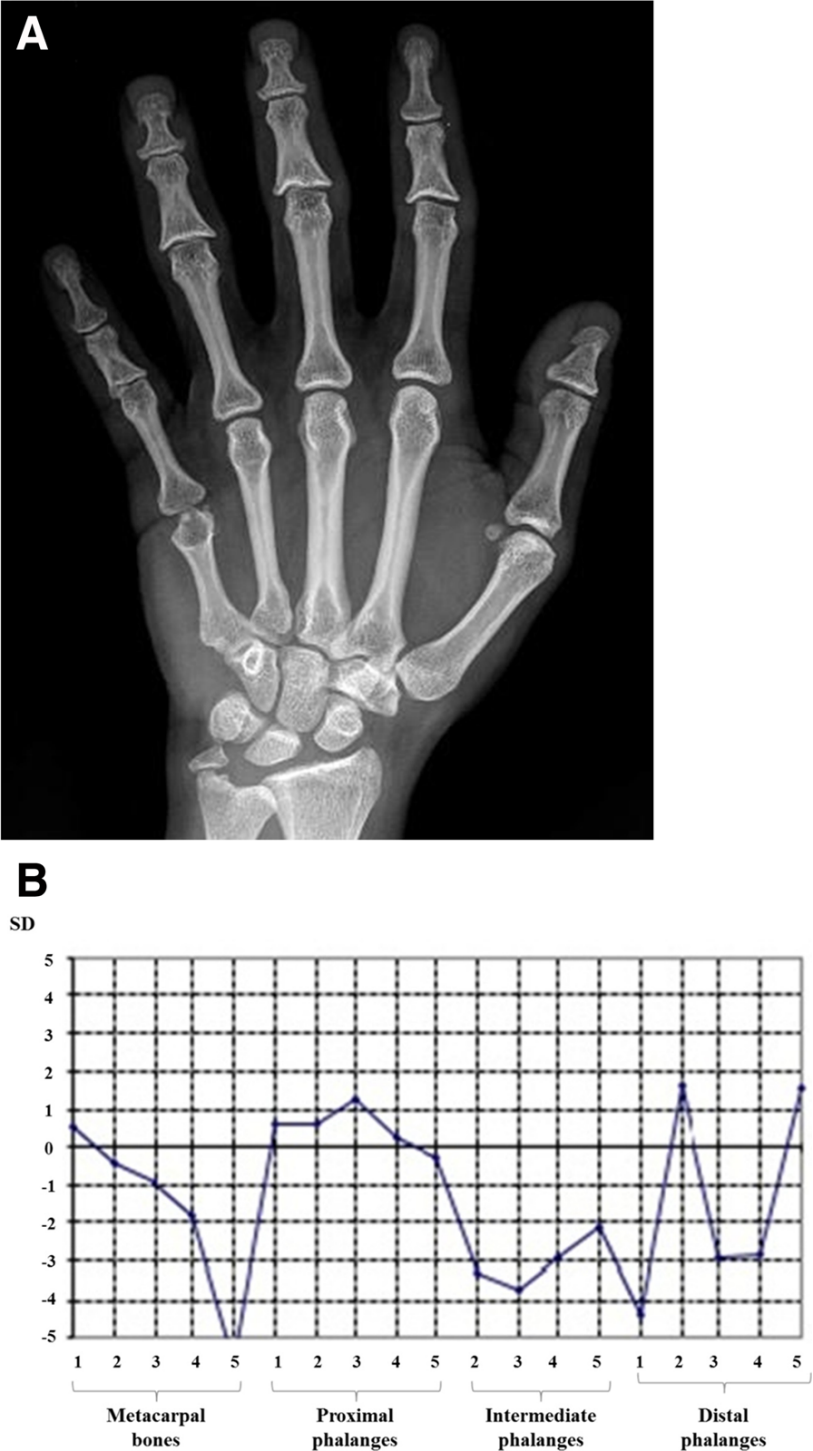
Radiograph of the mother’s left hand with morphologic study. a Cone-shaped epiphyses of middle phalanges, clinodactyly of 2nd finger, and brachymetacarpalia of 5th metacarpal. b Morphologic radiographic study: several bone segments are below − 2. Standard Deviation (SD): particularly, the fifth metacarpal bone is below − 5 SD
